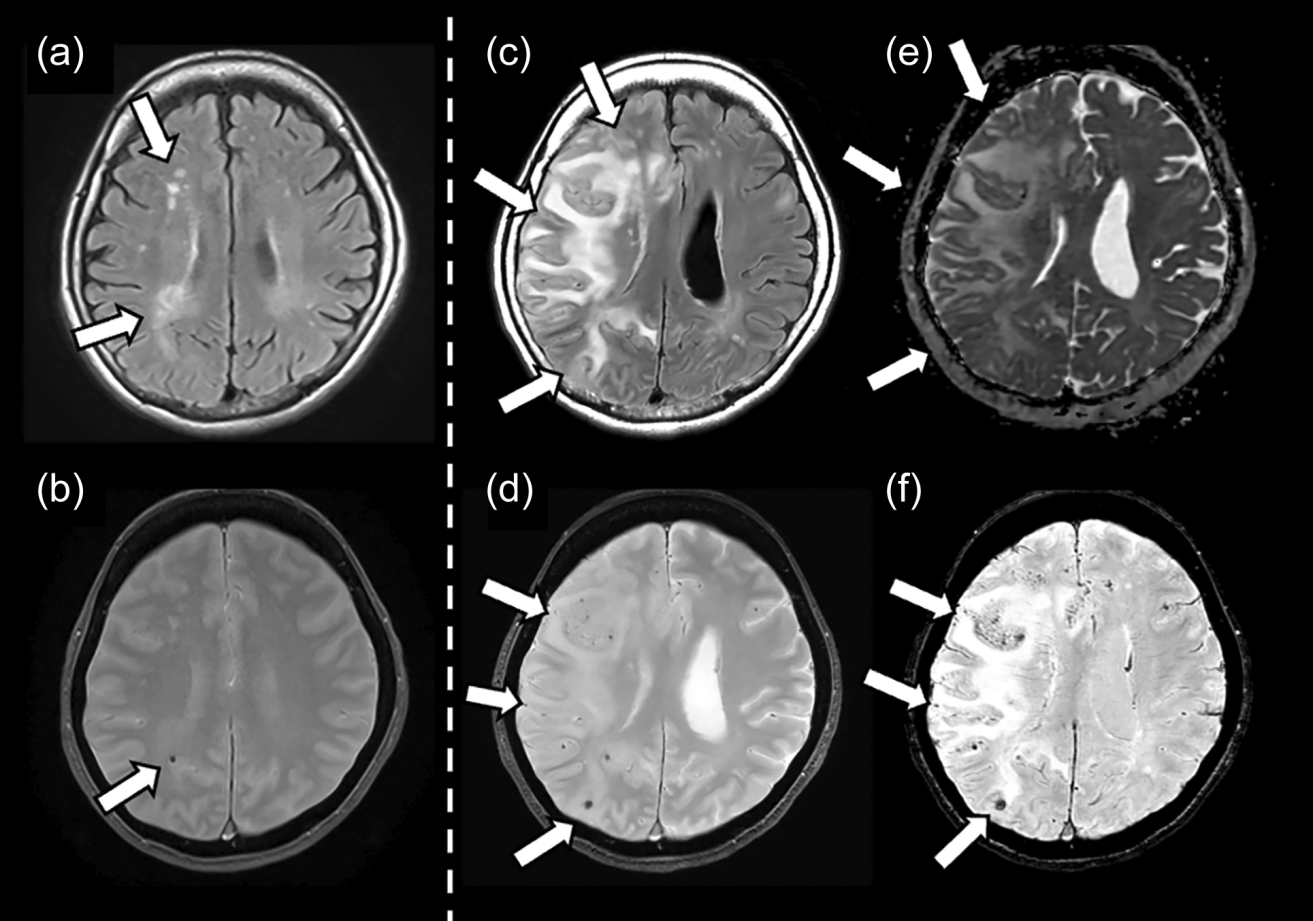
Magnetic resonance imaging (MRI) prior to treatment, and changes following treatment with lecanemab. Baseline MRI showing only subtle white matter hyperintensity on fluid‐attenuated inversion recovery (FLAIR). The white arrows show subtle white matter hyperintensity on FLAIR (a) and only two microbleeds on T_2_* weighted image (WI) (one of the two microbleeds is indicated by arrows in b, the white arrow shows microbleed). MRI taken 6 days following symptom onset showing extensive high‐signal areas in the white matter on FLAIR. The white arrows show extensive high‐signal areas in the white matter on FLAIR (c) and extensive microbleeds along the cortex and subcortical white matter on T_2_* WI. The white arrows show extensive microbleeds along the cortex and subcortical white matter on T2*WI (d). Consequently, the patient was diagnosed with severe amyloid‐related imaging abnormalities (ARIA) (E) and ARIA with haemorrhage (ARIA‐H) infections. The apparent diffusion coefficient map indicates increased diffusion. The white arrows indicate the presence of vasogeniv edema (e), indicating the presence of vasogenic oedema. Susceptibility‐weighted imaging (SWI), revealed numerous small microbleeds along the cortex. The white arrow show indicate new microbleeds (f), with more than 100 new microbleeds appearing throughout the brain. Despite numerous microbleeds, imaging findings did not indicate any superficial siderosis (data not shown).
